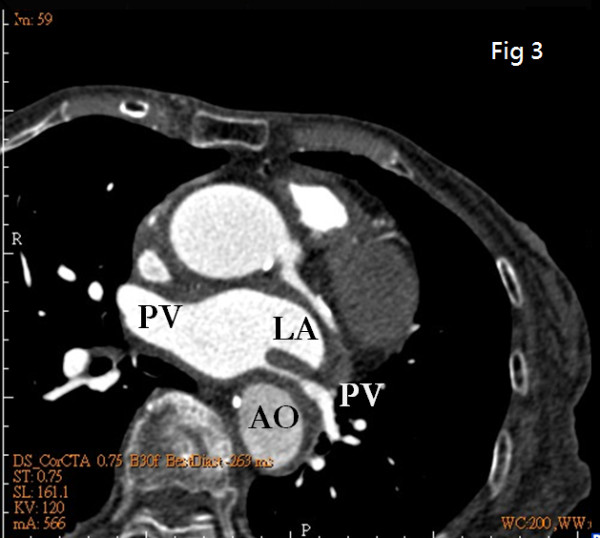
Another plane of CT images: the left pulmonary vein critically squeezed is clearly demonstrated.
